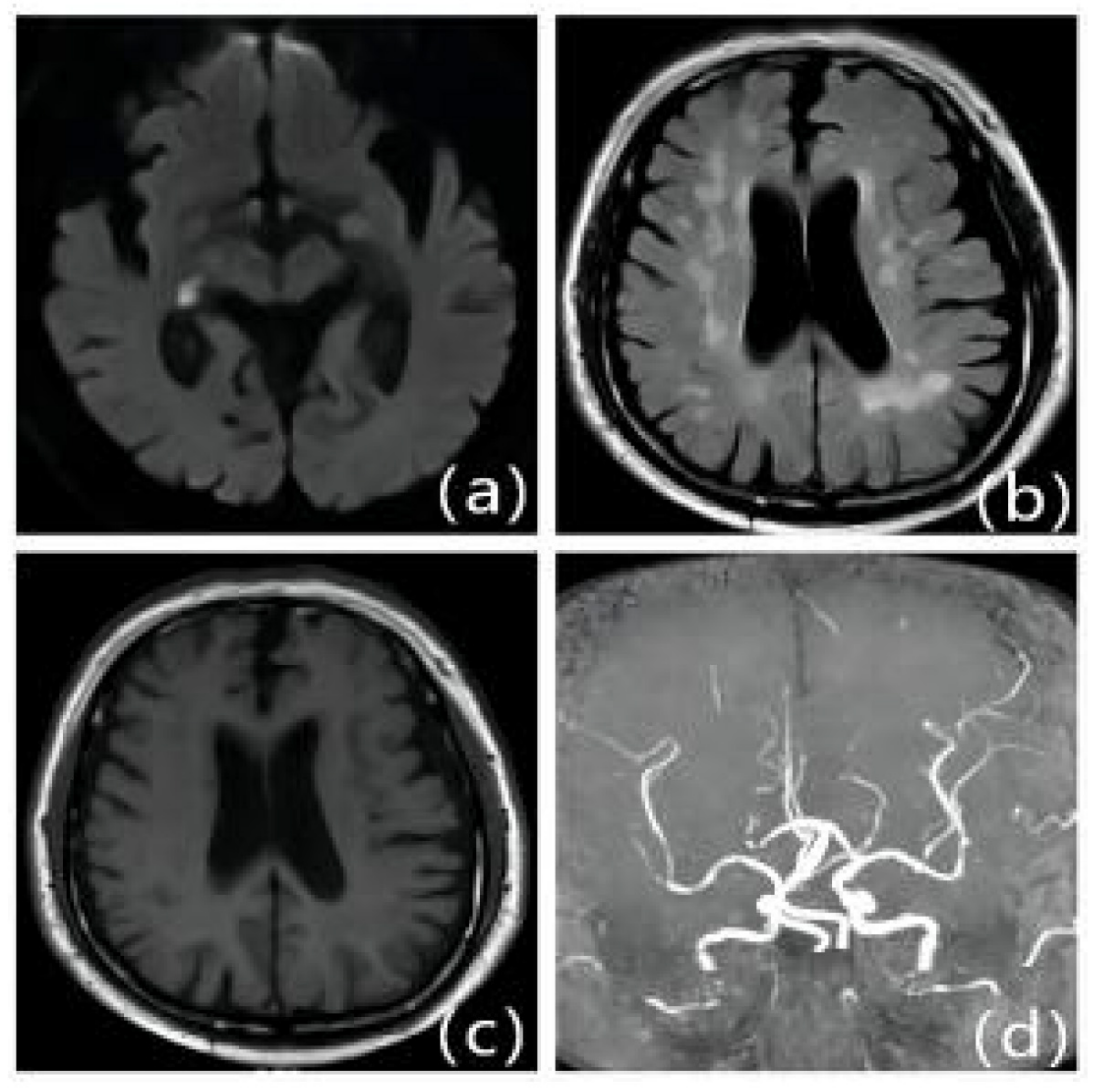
Patients with first-onset IS due to ICAS with severe LA: Male, 70 years old, admitted 9 hours after onset. A: DWI, B: T2WI-FLAIR, C: T1WI, D: MRA. DWI shows right thalamic lamellar cerebral infarction; MRA shows fewer distal branches and narrowing of the right middle cerebral artery than the contralateral side. T2WI-FLAIR and T1WI show diffuse demyelinating changes in the white matter of the brain around the ventricles bilaterally.
